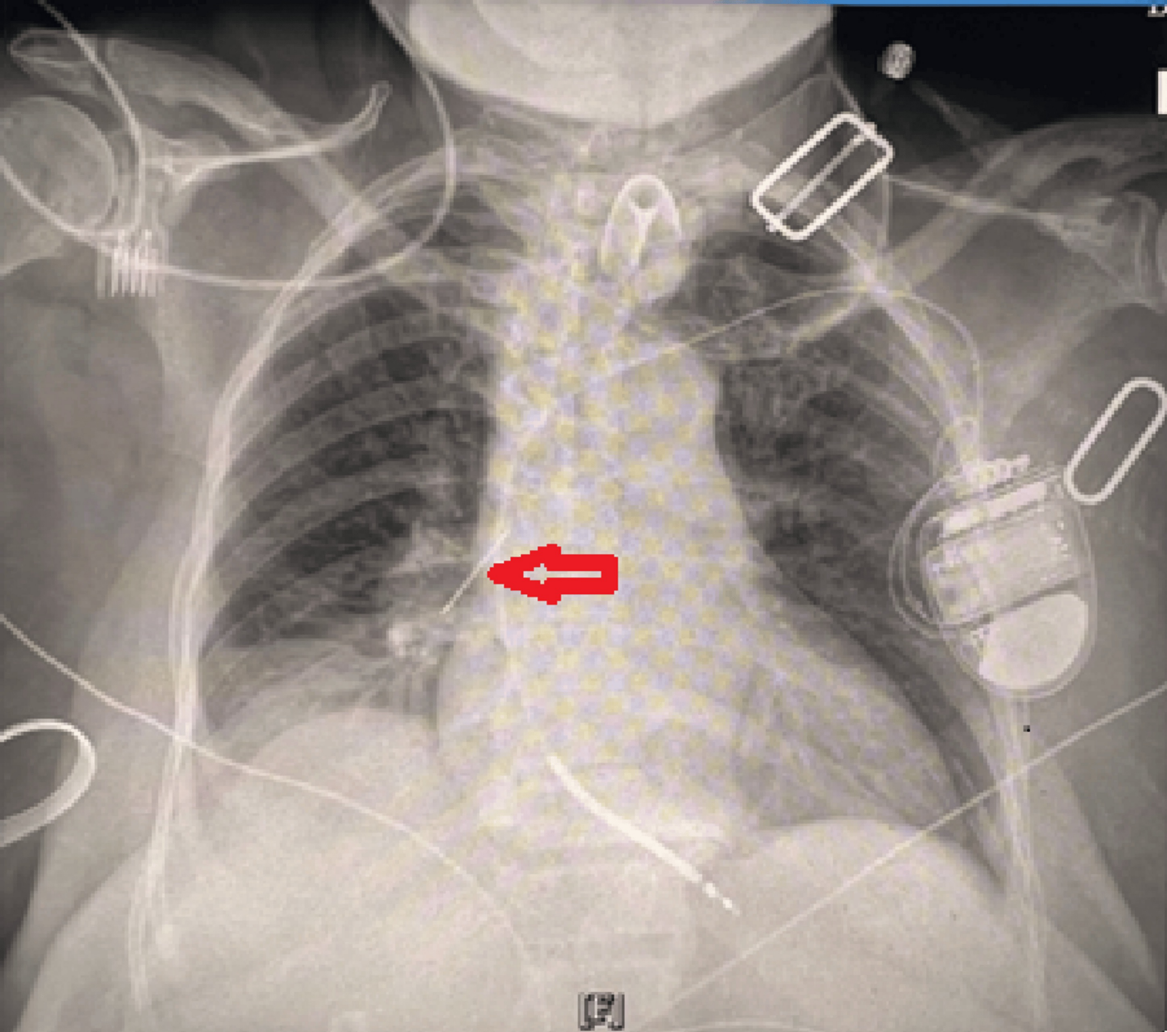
Portable AP chest radiograph showing left-sided ICD generator and right ventricular lead ICD: Implantable cardioverter defibrillator; A thin linear radiopaque density projects over the right hilum, with right basilar atelectasis and a small right pleural effusion.
